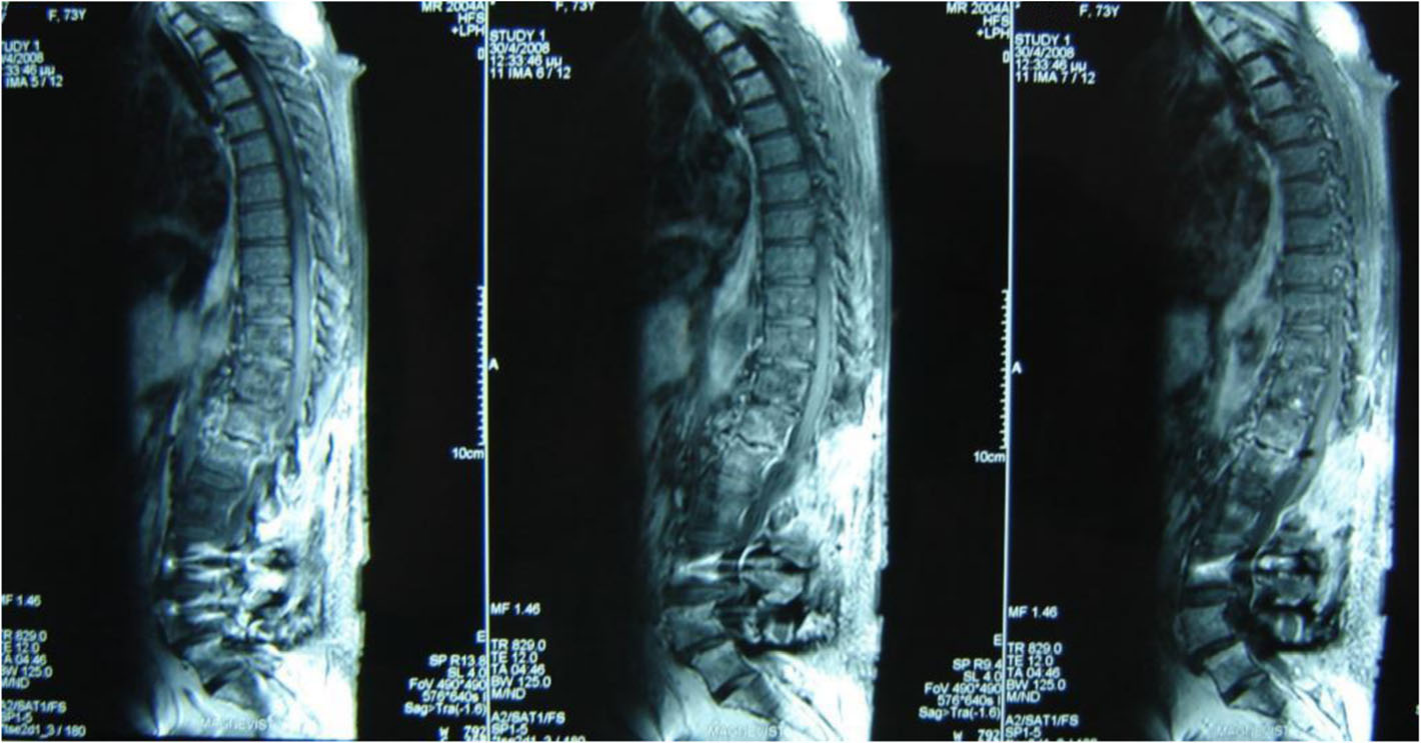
Magnetic resonance imaging showing signs of osteomyelitis at T12 and L1 vertebrae
